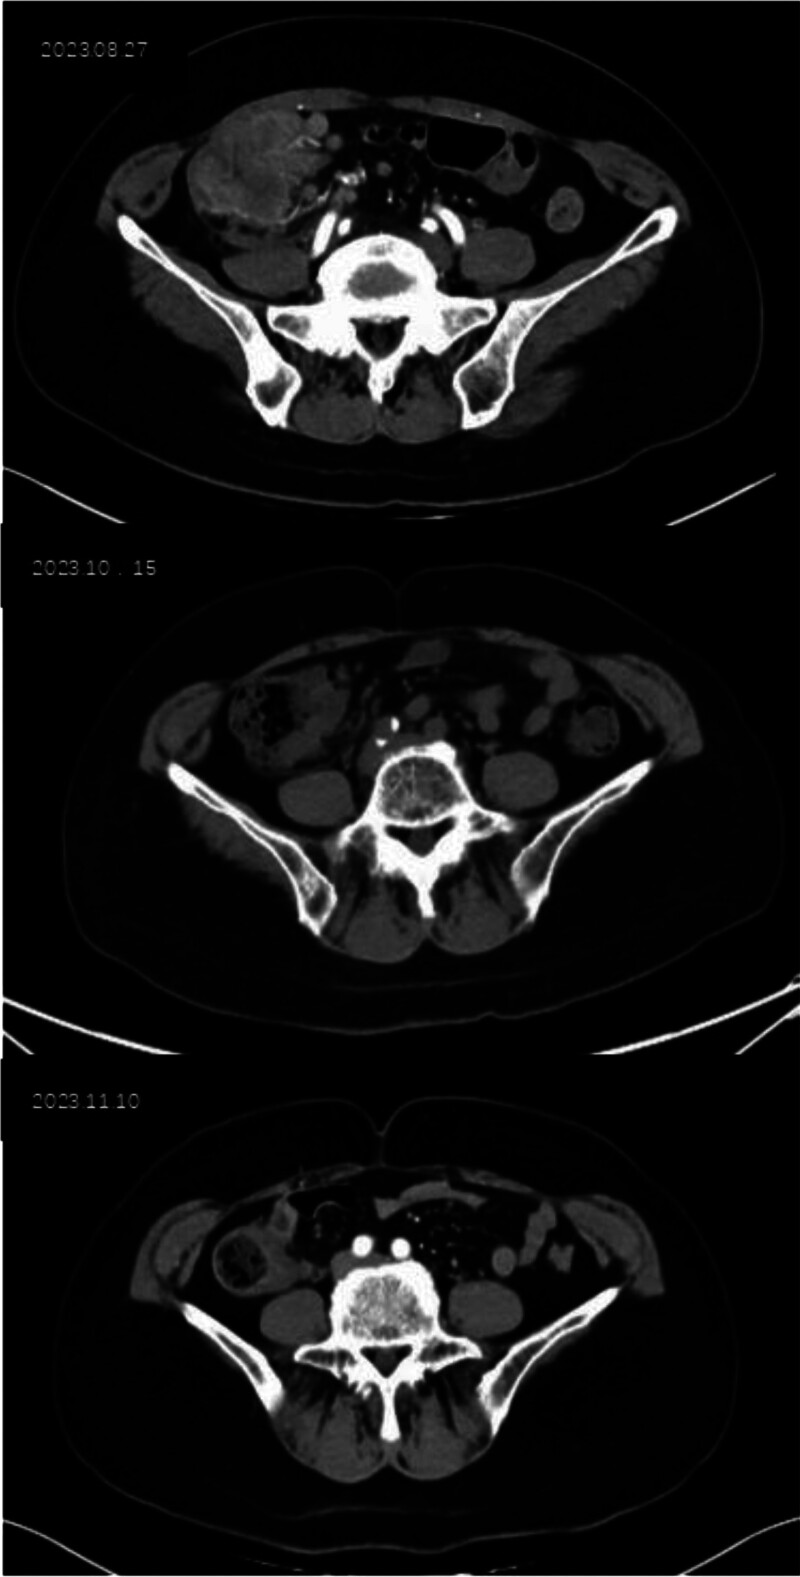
CT scans of the abdomen show changes in maximum tumor diameter and the mesenteric lymph nodes over time. CT = computed tomography.
